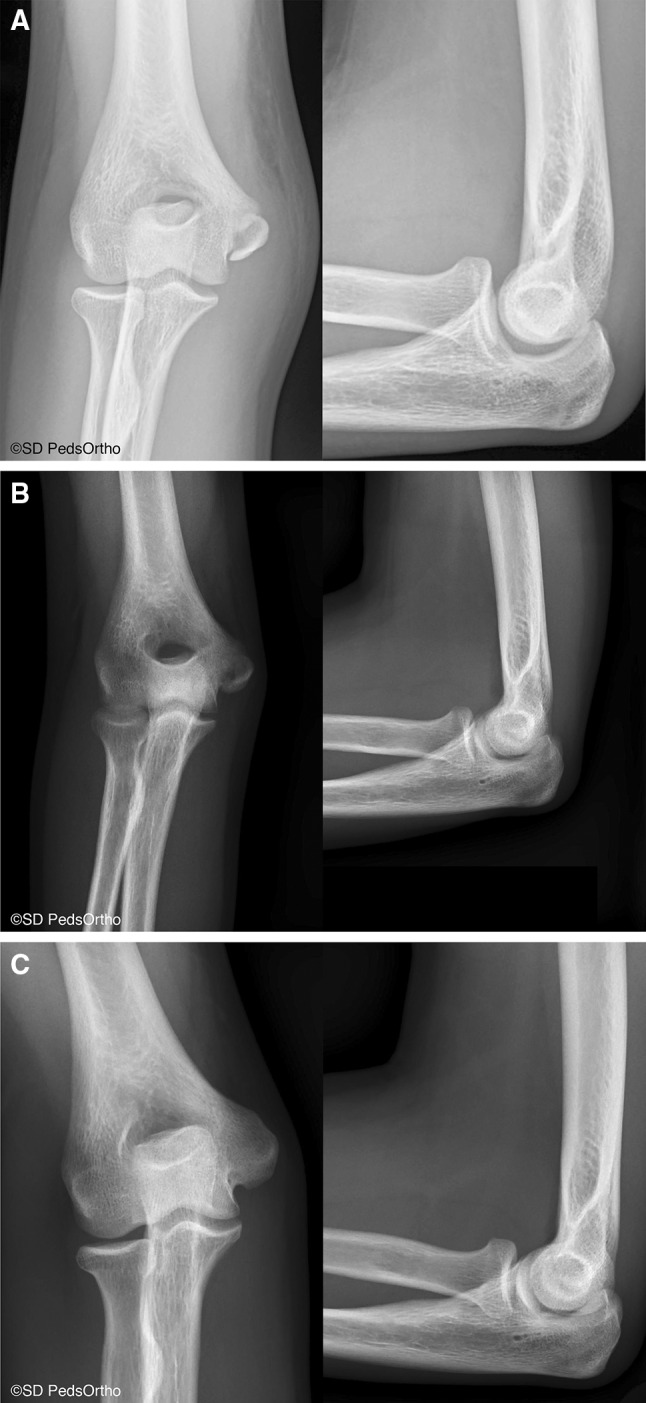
a Anteroposterior and lateral radiographs of a 15-year-old, right hand dominant, male, water polo player with an isolated right medial epicondyle fracture. b Two months postinjury, the fracture showed interval healing, and the patient was asymptomatic. c 1.5 years postinjury, the patient’s fracture had healed with a mild malunion that was asymptomatic and he returned to full sporting activities
